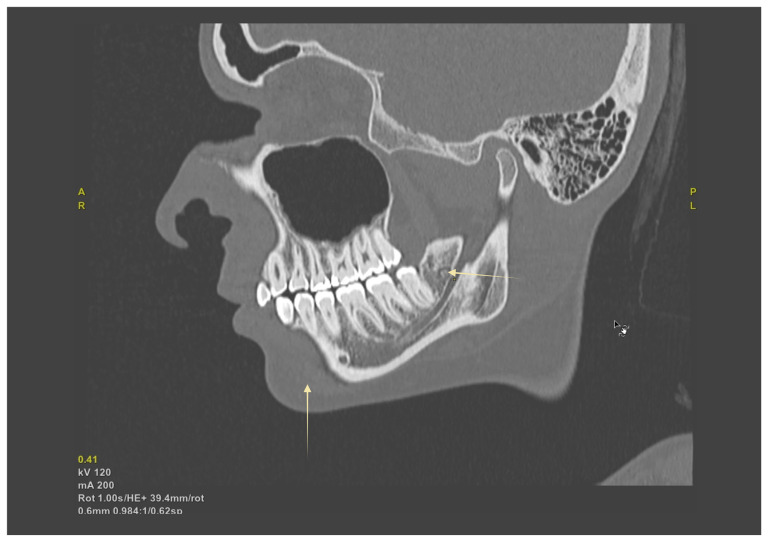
Oblique-sagittal CBCT reconstruction demonstrating the course of the mandibular canal (MC). The yellow arrows indicate the canal trajectory within the mandibular body and its proximity to the molar roots, serving as key landmarks for preoperative assessment and surgical planning.
